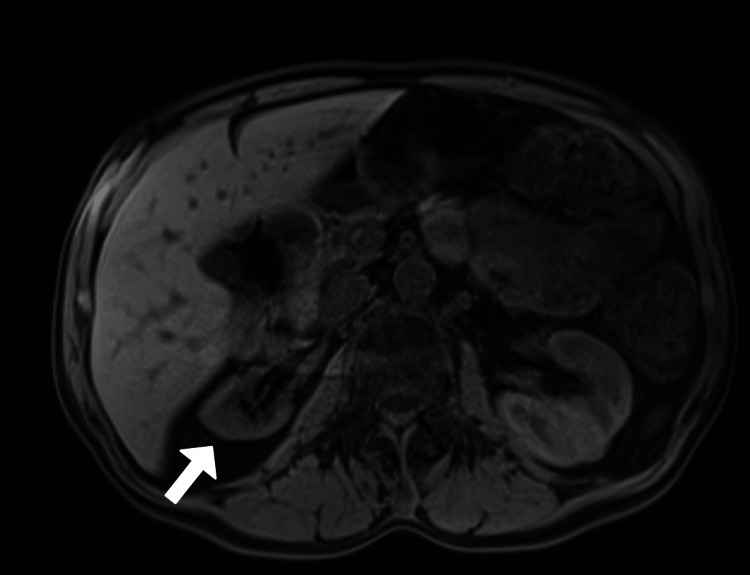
Axial T1 non-contrast MRI of the abdomen, demonstrating the patient's atrophic right kidney
